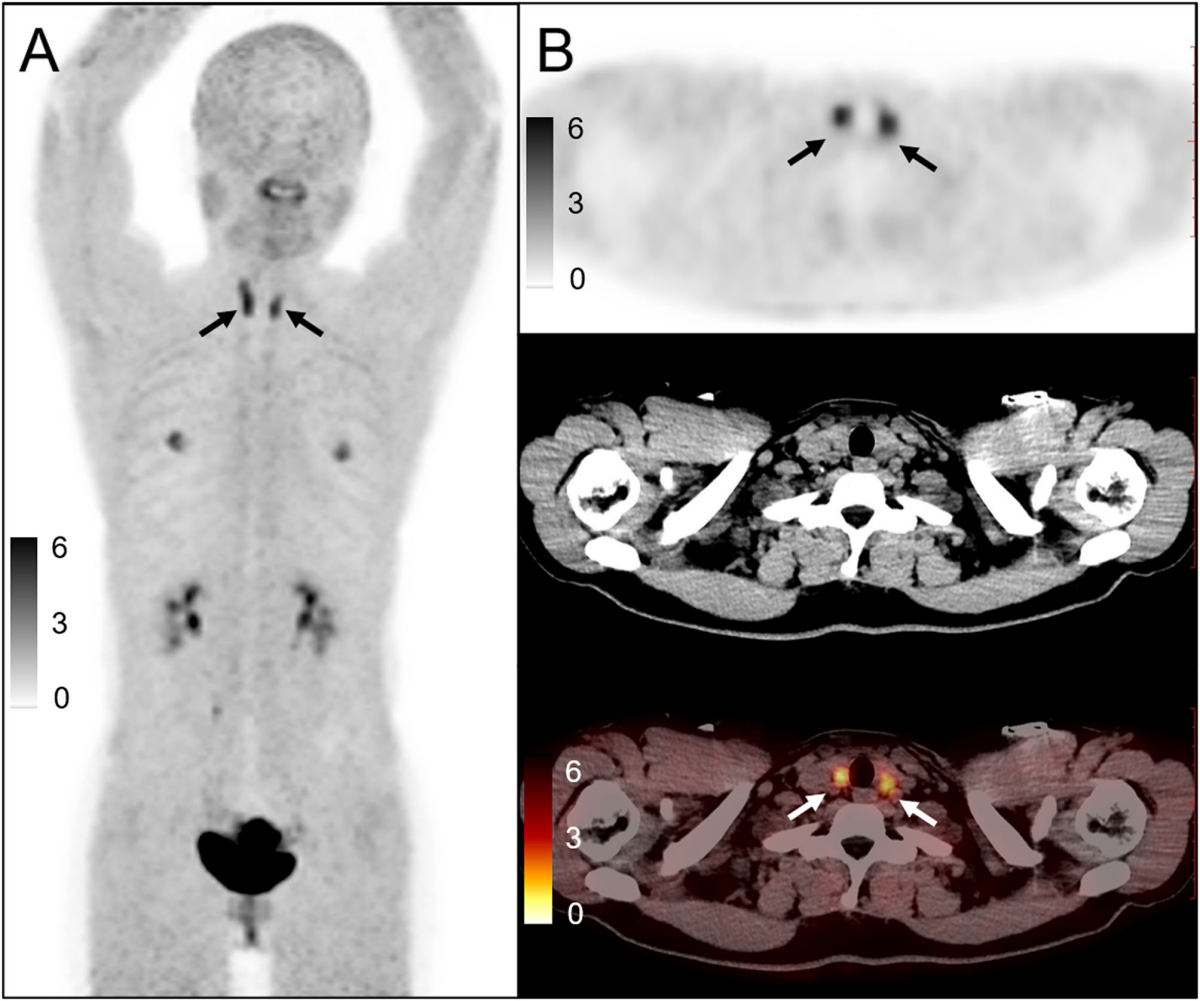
A 32-year-old woman underwent ^68^Ga-FAPI PET/CT for rectal cancer after surgery [(A): maximum intensity projection image; (B): upper, PET, middle, CT, lower, PET/CT image]. The uptake of thyroid ^68^Ga-FAPI increased diffusedly, and the SUVmax was about 4.6, and TBR was 7.54. CT showed a decrease in density. Ultrasound examination showed that the echo of the thyroid gland was reduced and the blood flow was uneven. A fine needle aspiration biopsy of the thyroid revealed chronic thyroiditis. Thyroid function test revealed abnormal TSH and thyroid antibody levels (TSH: 16.117, reference range, 0.38–5.57 mIU/L; FT3:2.07, reference range, 1.80–3.80 pg/ml; FT4: 0.86, reference range, 0.78–1.86 ng/dl; TPOAb: 872.18, reference range, 0.0–2.6 IU/ml; TgAb: 620.47, reference range, 0.0–14.58 IU/ml), conforming the diagnosis of lymphocytic thyroiditis.
